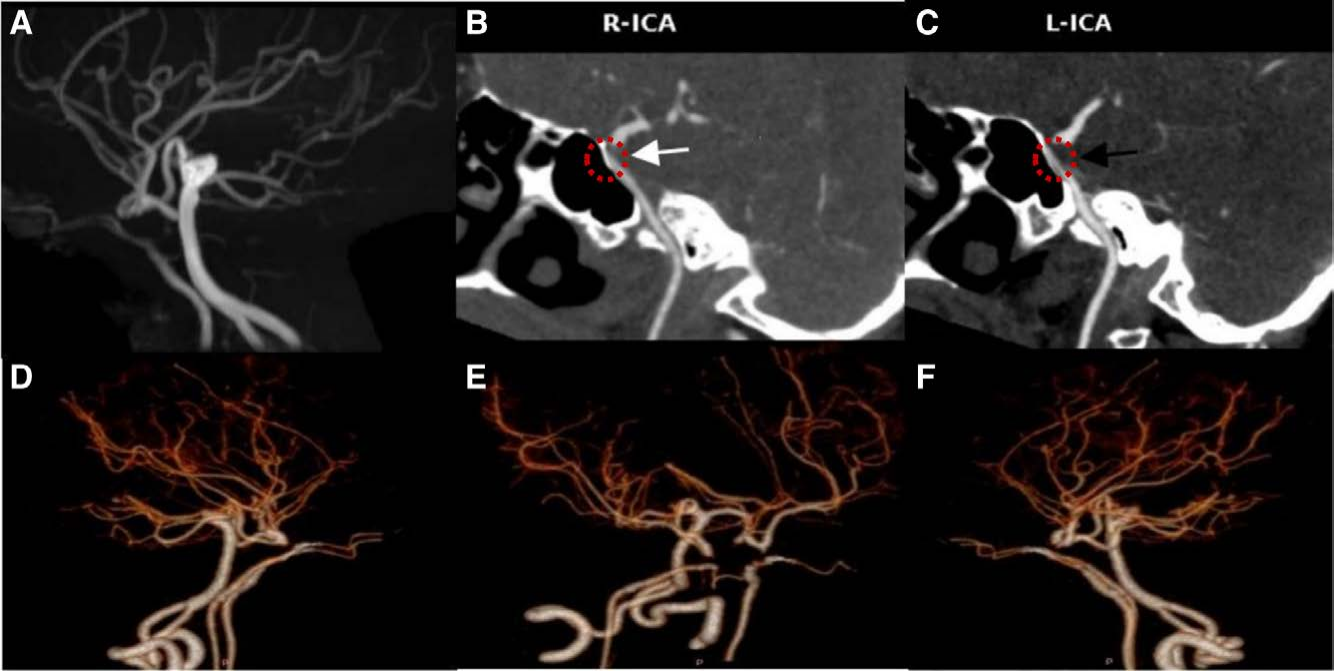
MRA and CTA images of the patient. (A) Head MRA shows simultaneous absence of the eye segments and communicating segments of the bilateral internal carotid arteries. (B) CTA shows absence of the right internal carotid artery’s ophthalmic segment and communicating segment (white arrow with red dashed circle). (C) CTA shows absence of the left internal carotid artery ophthalmic segment and communicating segment (black arrow with red dashed circle). (D–F) CTA shows that the bilateral ophthalmic arteries continue to the distal segments of the bilateral internal carotid arteries, with simultaneous absence of the ophthalmic and communicating segments of the bilateral internal carotid arteries, tortuosity of the basilar artery, and patency of the bilateral posterior communicating arteries. CTA = computed tomography angiography, MRA = magnetic resonance angiography.
